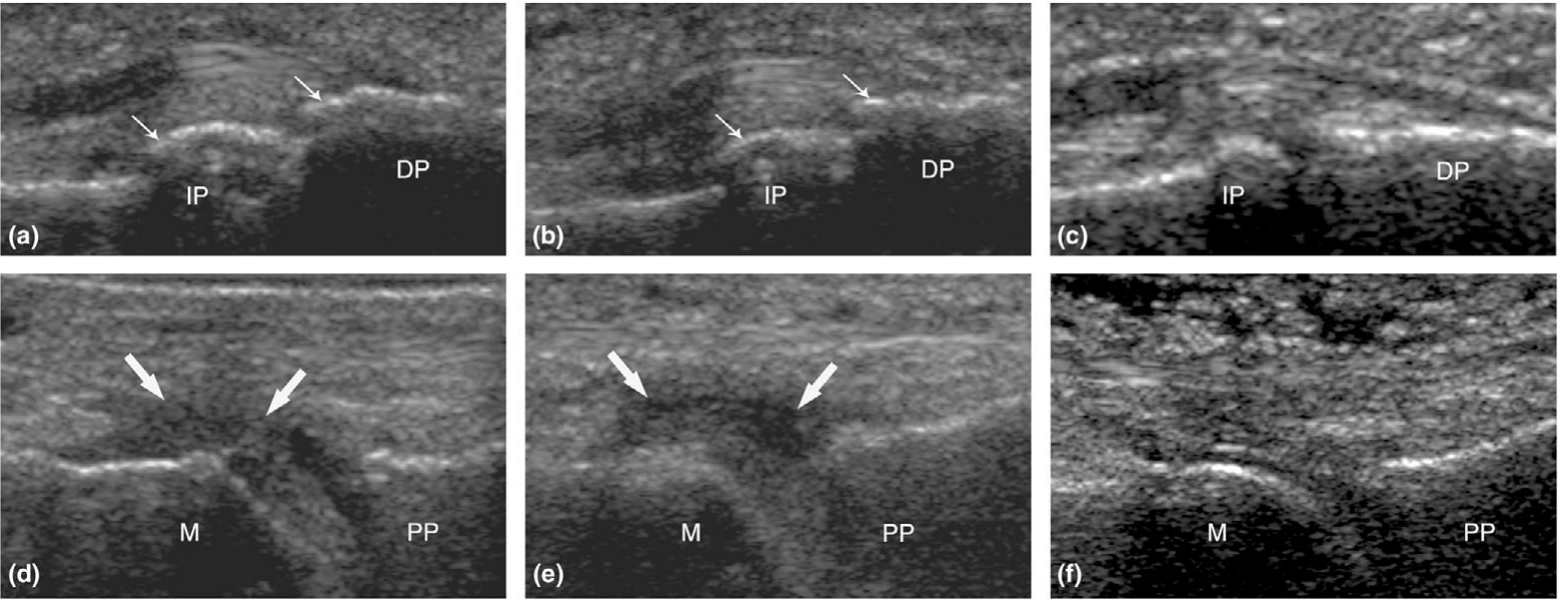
Ultrasonography (US) of distal interphalangeal (DIP) joints (a-c) and metatarsophalangeal (MTP) joints (d-f). Images on the left were acquired independently by ultrasonographer 1 (Charlotte Wiell) and middle images were acquired independently by ultrasonographer 2 (Marcin Szkudlarek) in the interobserver US substudy. (a,b) Bone proliferations (arrows) in the 2nd DIP joint on US in a palmar view in a patient with psoriasis-associated arthritis (PsA). (d,e) Synovitis (arrows) in the 2nd MTP joint on US in a dorsal view in a patient with PsA. Images on the right show a 2nd DIP joint (c) and a 2nd MTP joint (f) without destructive or inflammatory changes on US. (f) Notice subcutaneous edema dorsal to the 2nd MTP joint. DP, distal phalanx; IP, intermediate phalanx; M, metatarsal bone; PP, proximal phalanx.
